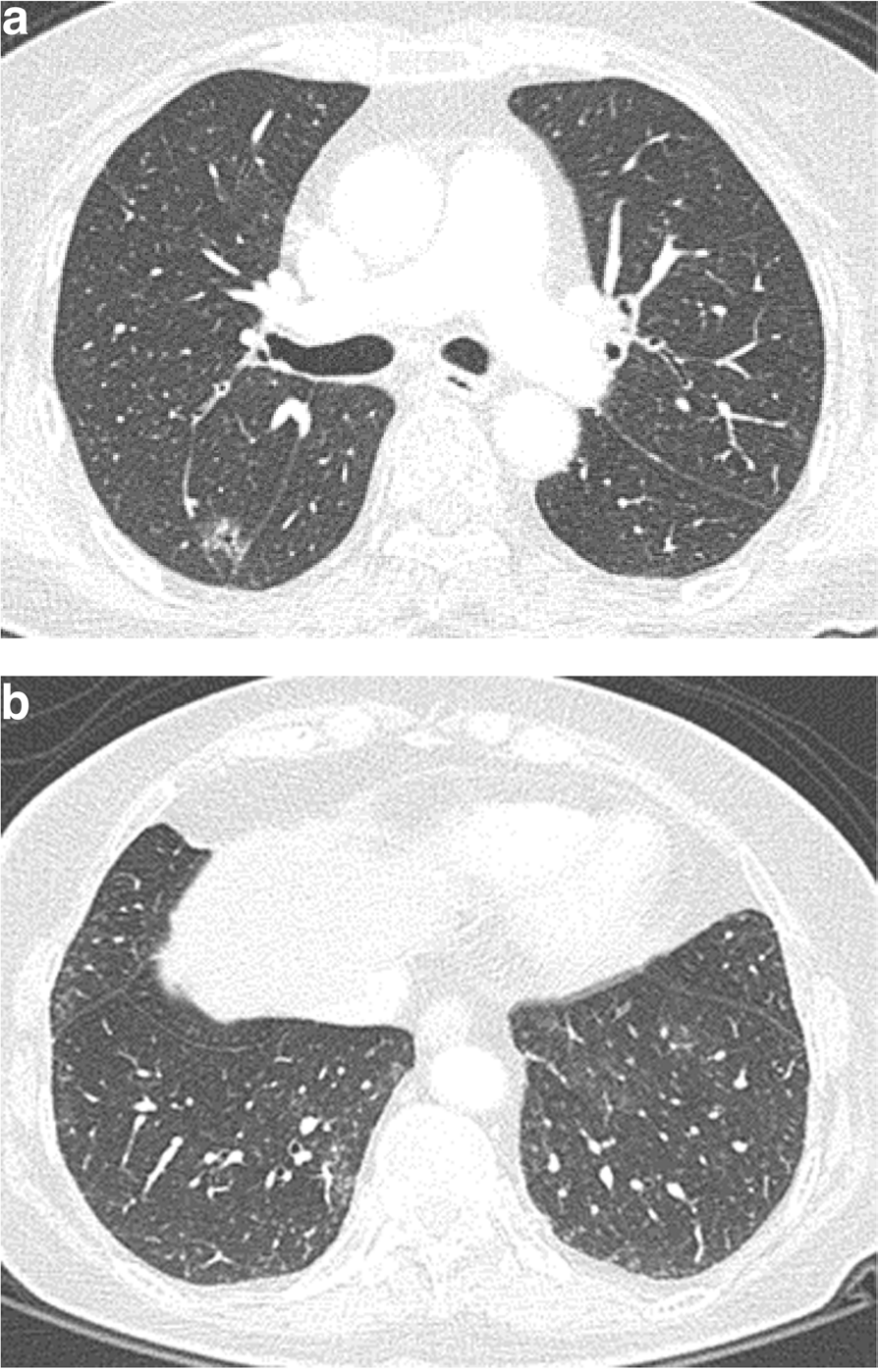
A 69-year-old female nonsmoker with stage I adenocarcinoma located in the right upper lobe. Pre-treatment chest CT demonstrated the tumor with signs of lobulation, spiculation, vacuole and pleural traction (a). Ground-glass opacity and reticulation were observed in bilateral lower lobes without subpleural predominance, in accordance with non-fibrotic ILA (b). The patient underwent surgery, and a pathological diagnosis of adenocarcinoma with EGFR mutation was made. She was still alive at the end of follow-up. CT, computed tomography
